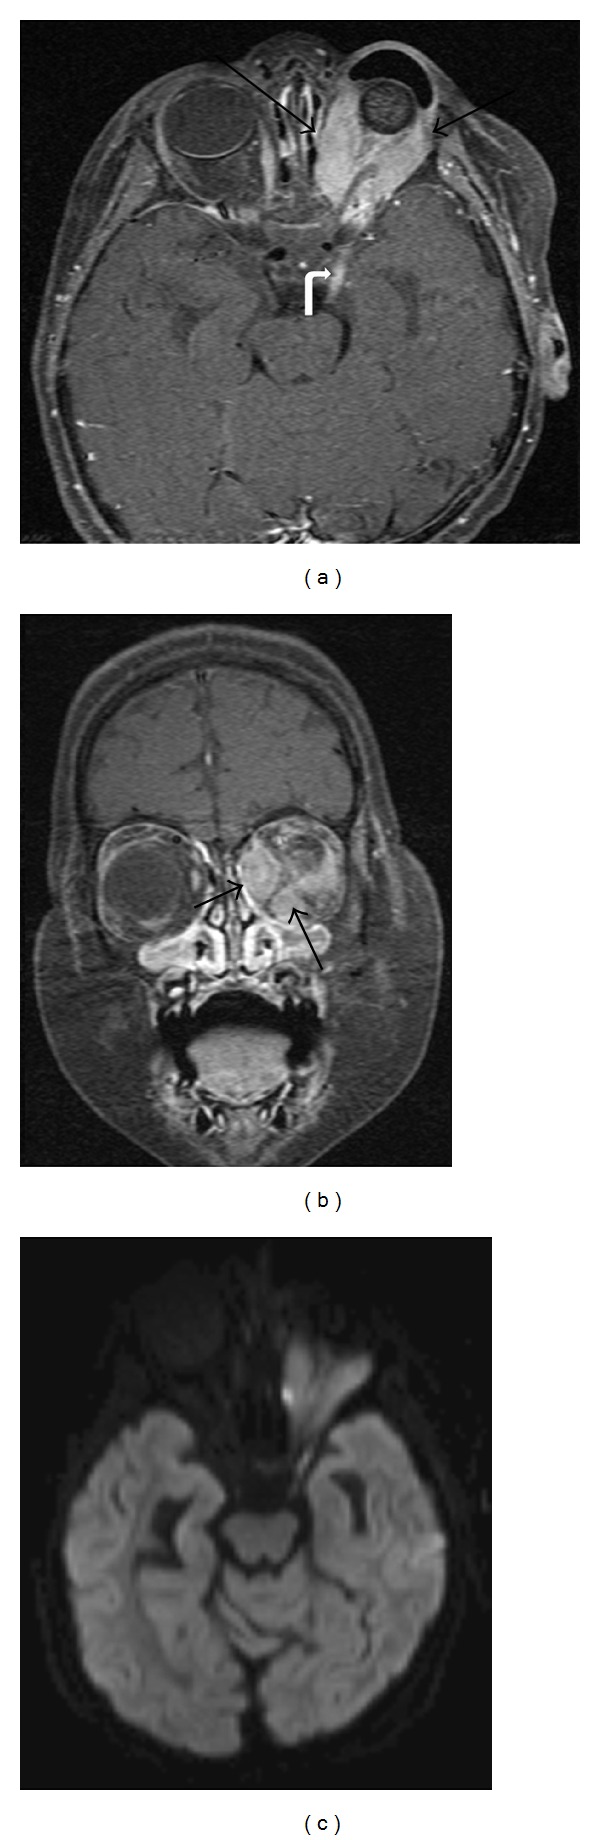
8 years old with orbital prosthesis status postenucleation for retinoblastoma presents with proptosis. MR axial (a) and sagittal (b) T1-weighted postcontrast fat-saturated images demonstrate homogeneously enhancing infiltrative tissue behind the prosthesis (black arrows). Increased signal along the course of the optic nerve is consistent with perineural spread of disease (curved white arrow). Axial diffusion-weighted image at the same level shows increased signal within the mass due to highly cellular tissue (decreased diffusion, corroborated by ADC maps (not shown)). Biopsy confirmed lymphoma.
